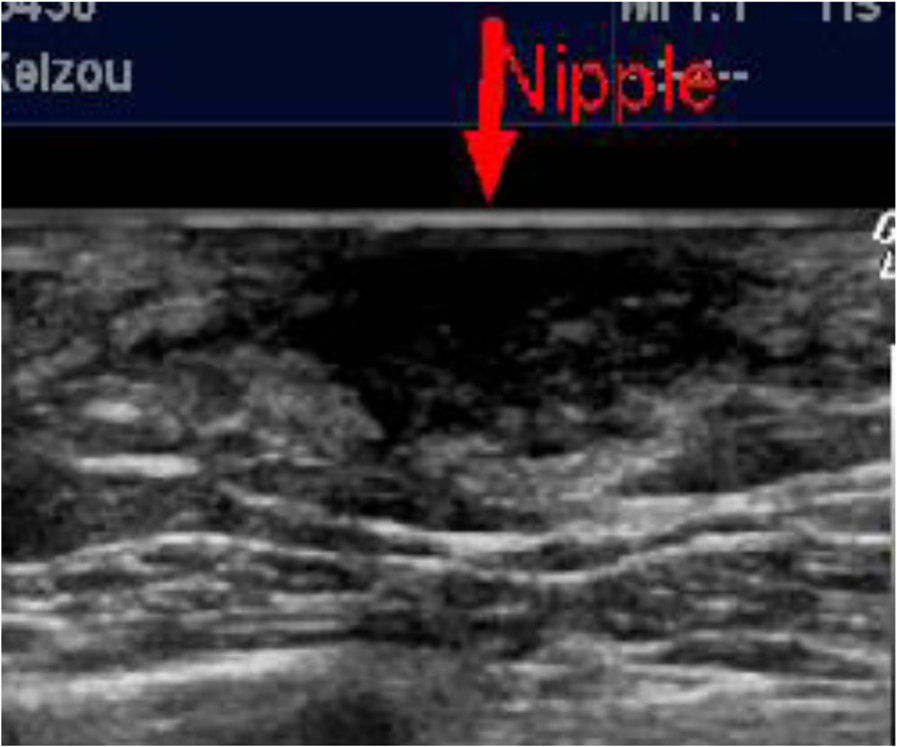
Mammary ultrasonography. A poorly marginated and low echoic area was detected under the nipple. The palpable mass was diagnosed as gynecomastia
